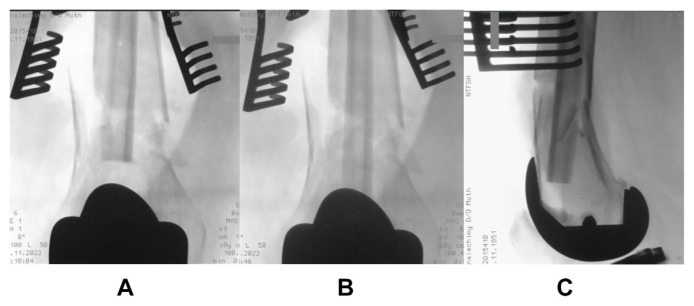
Intraoperative radiographs show the fibular strut allograft being inserted through the fracture site and distalized, helping to maintain length.
